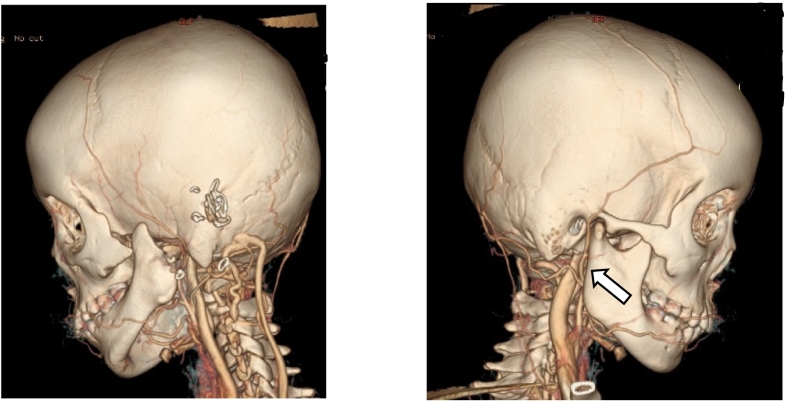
From the CT-Angiography there is a tortuosity of left vertebral vein with the diameter of the left is bigger than the right.
